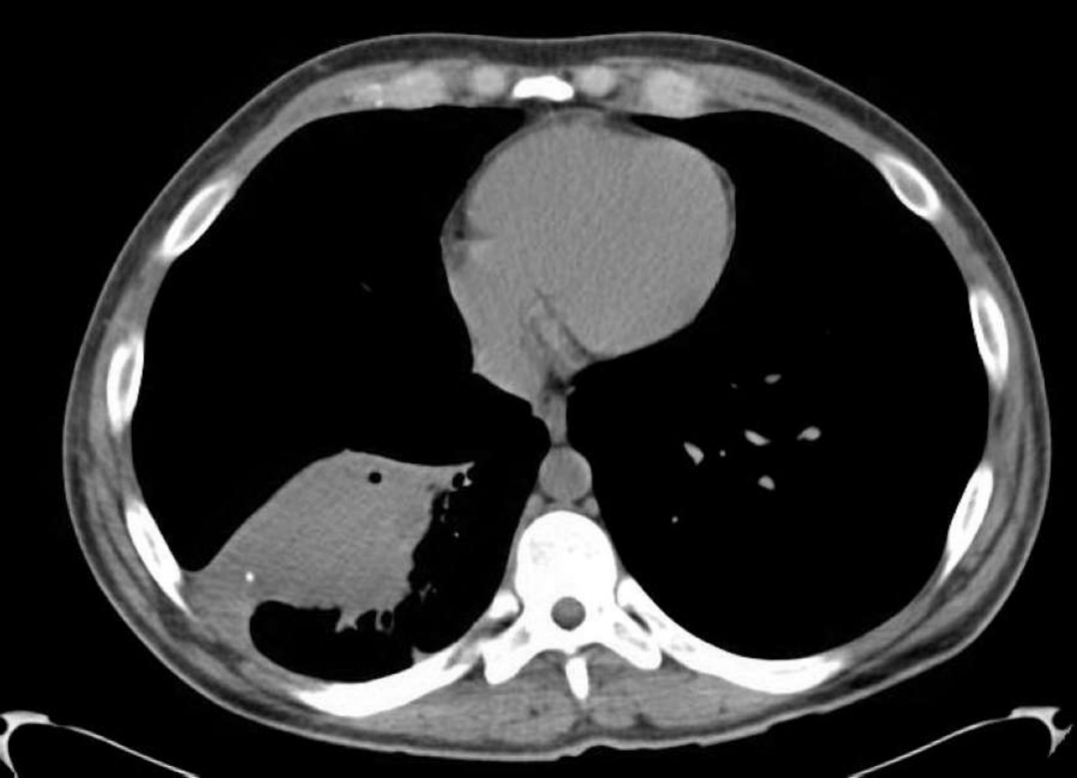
Mediastinal window of the chest CT. Irregular mass-like consolidation in the right lower lobe abutting the major fissure and diaphragmatic pleura, measuring at least 9.5 x 5.0 cm
